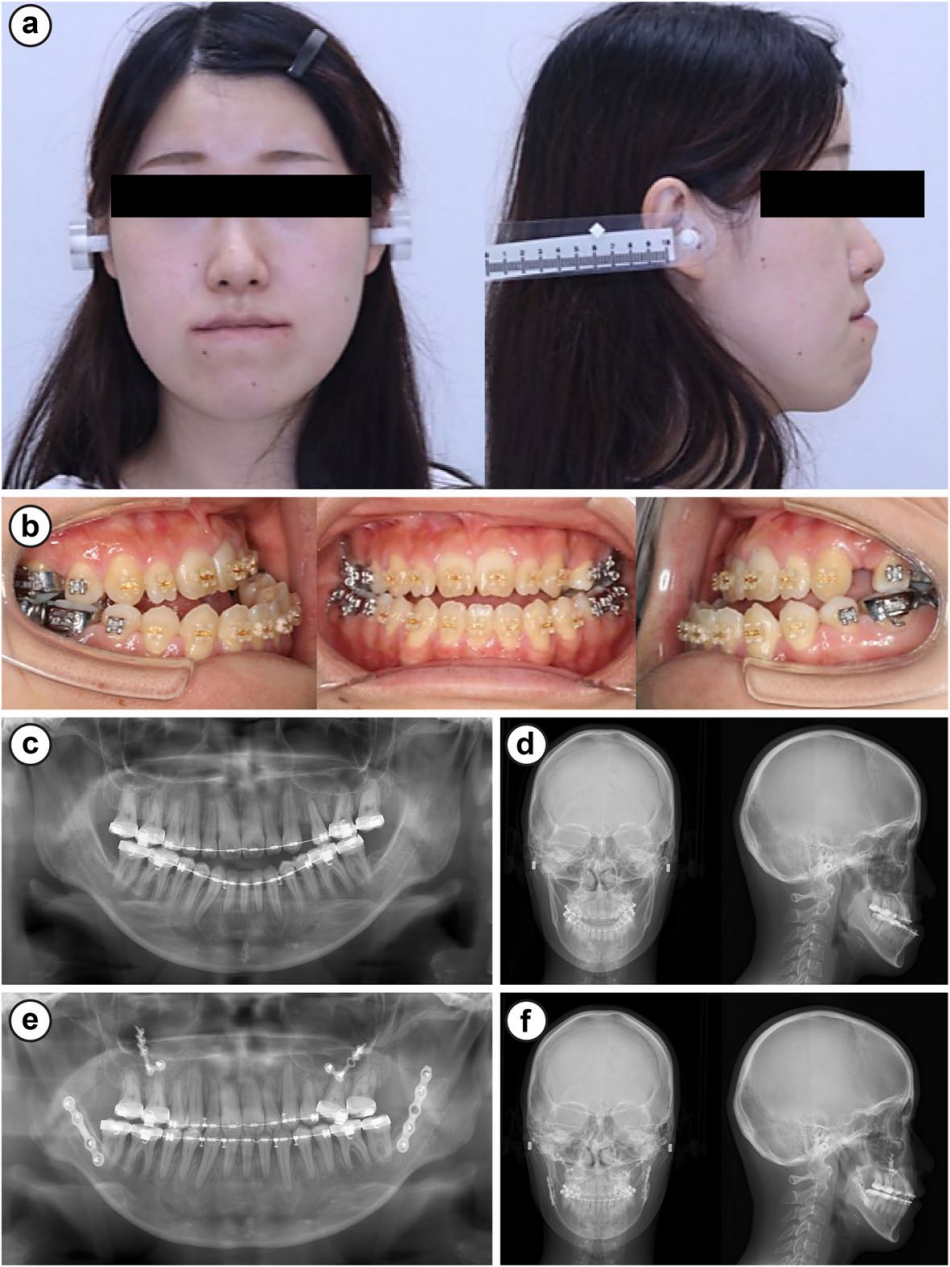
Case 10. a Facial photographs, b intraoral images, c panoramic radiographs, d cephalometric radiogram, e panoramic radiographs 1 year 6 months postoperatively, and f cephalometric radiogram 1 year 6 months postoperatively
